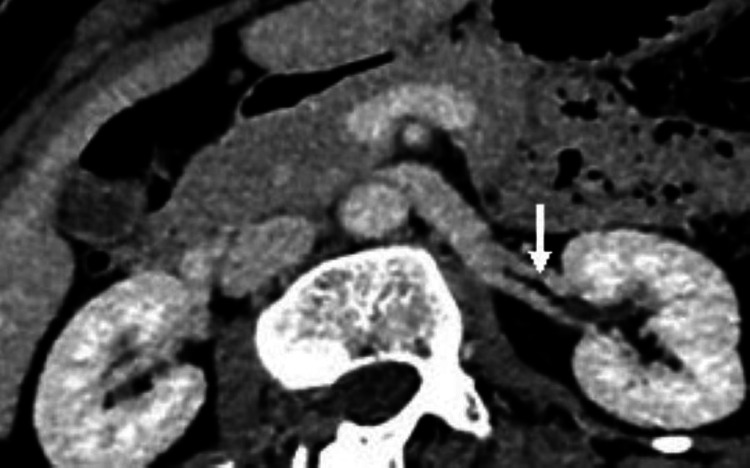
Nephrographic phase of CT angiography shows late confluence of left renal vein.
